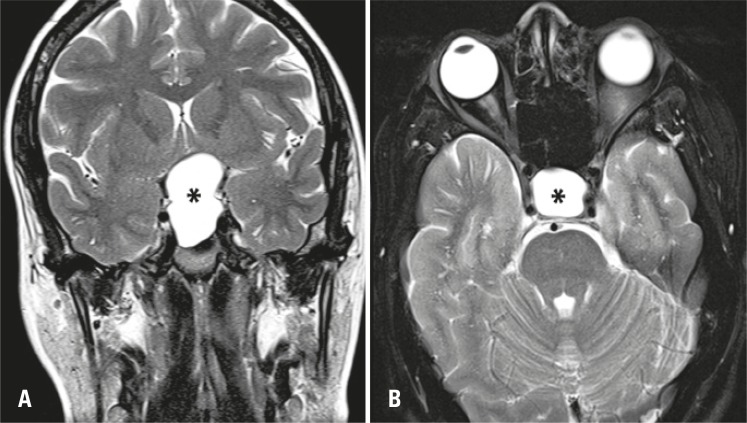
T2-weighted magnetic resonance images. (A) Coronal view revealing a large hyperintense and well-delineated sellar lesion (asterisk) with significant suprasellar extension. (B) Axial view. The homogeneous lesion (asterisk) occupies the whole sella turcica
